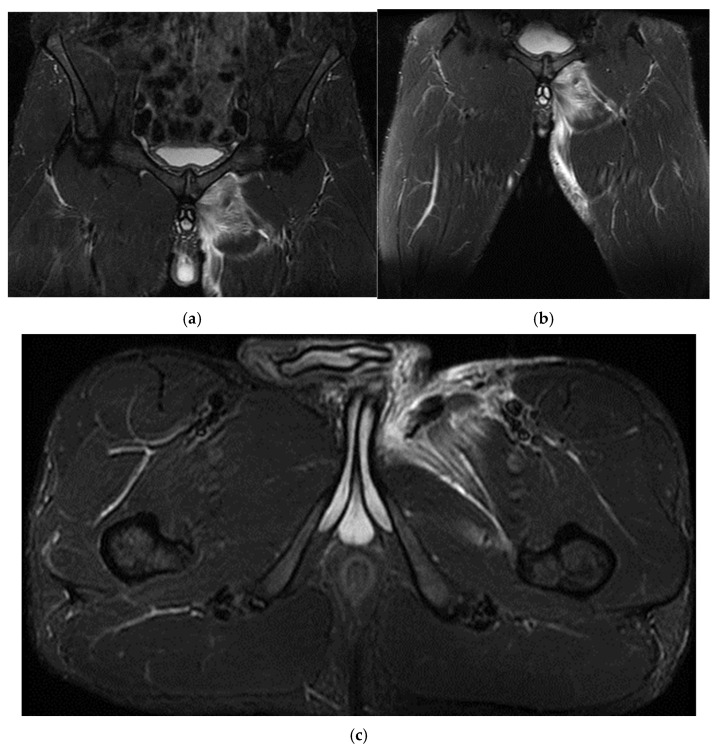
(a) Thigh MRI: coronal stir acquisition, (b) thigh MRI: coronal stir acquisition, (c) thigh MRI: axial stir acquisition.
